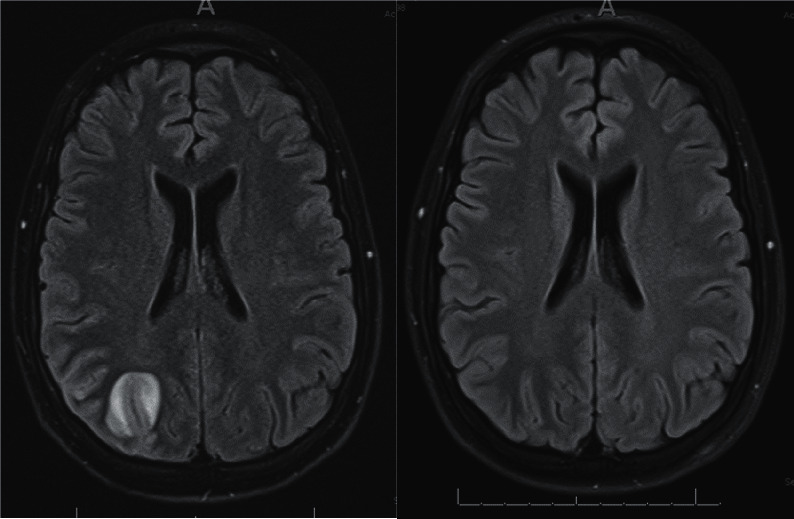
MRI brain at time of relapse (August 2019), on the left, demonstrating a 3.4 cm enhancing lesion within the parietal region with associated leptomeningeal enhancement. On the right, a repeat MRI brain (January 2020) after 3 months on alectinib monotherapy showing resolution of lesion and leptomeningeal enhancement.
